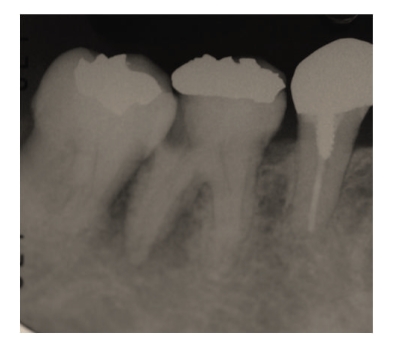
Case 2: Radiography at the first visit showing radiolucency at the apex area of tooth 46.
